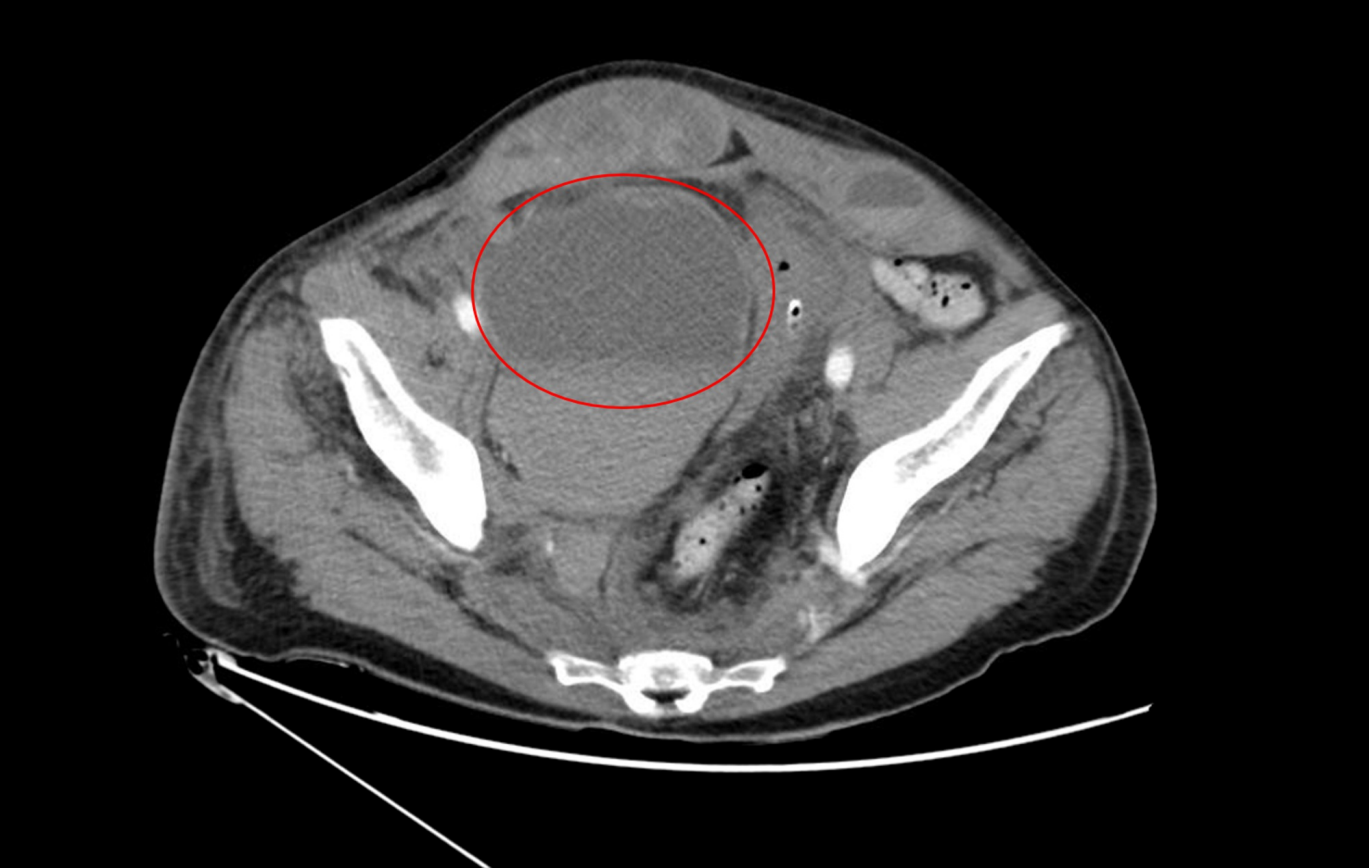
Contrast-enhanced CT pelvis (axial) showing right-sided rectus sheath hematoma (red circle) with extension into the right paracolic gutter.
